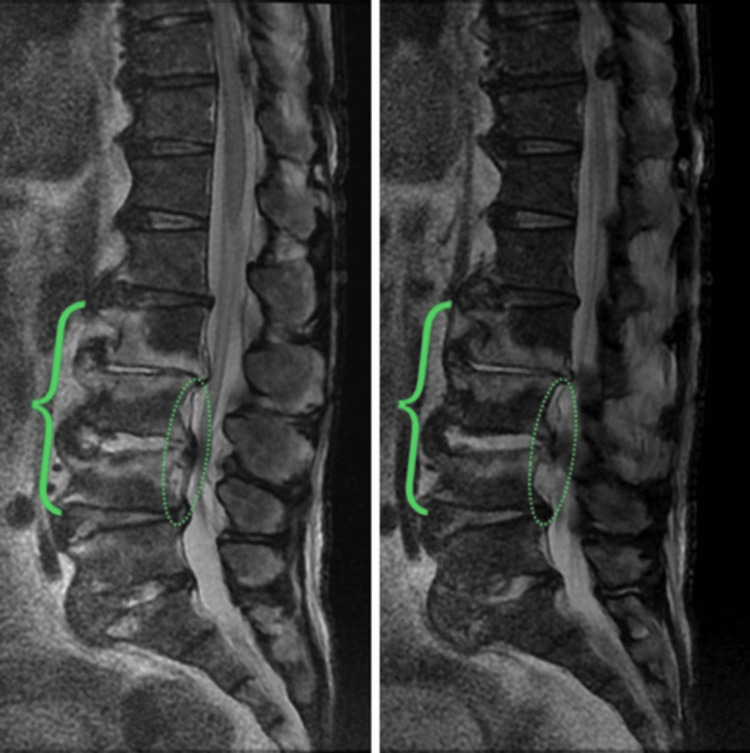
Vertebral magnetic resonance imaging (MRI) on day 25: T2-weighted image (T2, left) and T2-weighted image with frequency-selective fat suppression (T2FS, right). MRI T2 and T2FS showed high intensity of L2-L4 (the bracketed) vertebrae suggesting osteomyelitis and high intensity area posterior to the L3-L4 vertebrae (the circled areas) suggesting an epidural abscess.
